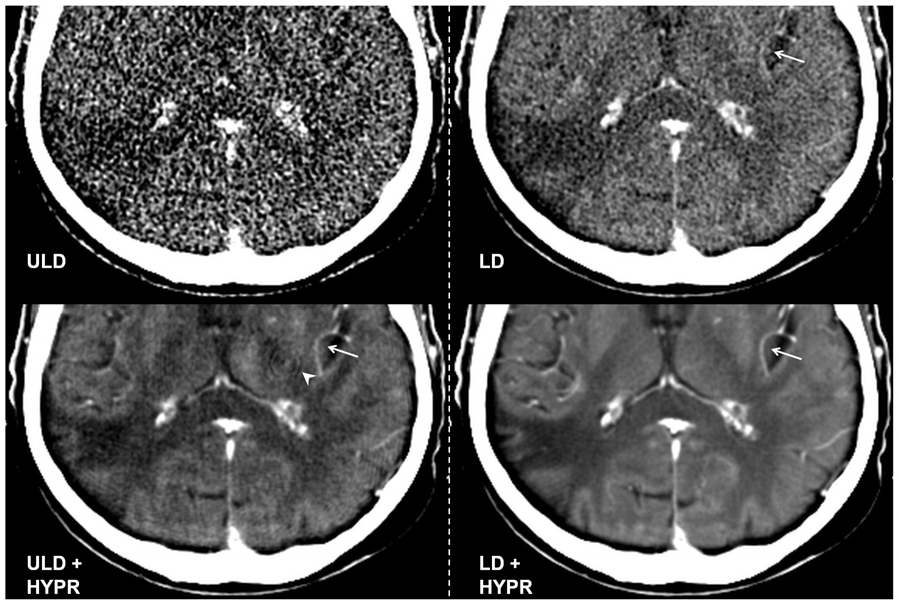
Ultra Low Dose (ULD), HYPR-LR-post-processed ULD (ULD+HYPR), Low Dose (LD) and HYPR-LR-post-processed LD (LD+HYPR) brain perfusion CT of a 68-year old male patient with a chronic infarction in the posterior territory of the right MCA (detail view). All images acquired at the same time point (last image of the 60 s time series). The ULD image was rated non-diagnostic (5), the infarction is not clearly visualized, details like small vessels (arrow in the other images) can not be clearly identified due to high image noise. Ring artifacts, which were present but not recognizable in the noisy ULD image are recognizable after HYPR post-processing (arrowhead in ULD + HYPR). Despite the artifacts, the subjective image quality was rated equal in ULD+HYPR and LD and images (both classified as 3). Excellent subjective image quality (classified as 1) in the LD+HYPR image with good differentiation between gray and white matter.
